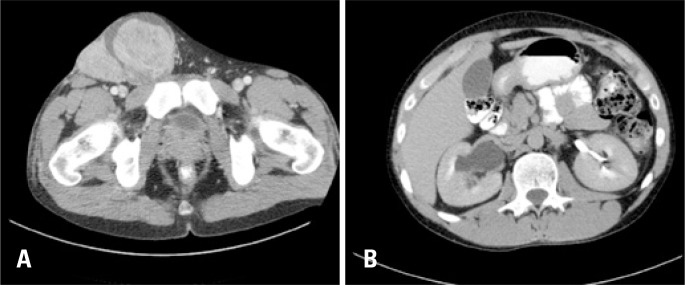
(A) Computed tomography showed a right testicular mass in contiguity with the ipsilateral inguinal canal; (B) hydronephrosis on the right due to compression by the scrotal mass that extended to the retroperitoneum
